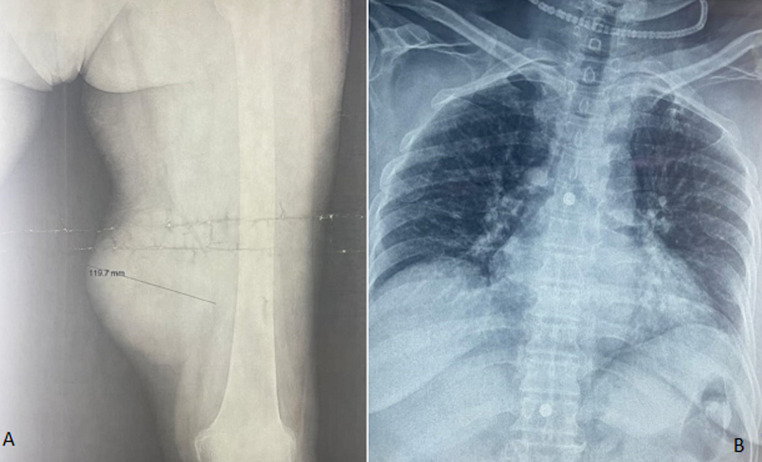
A) X-ray of the left thigh showing an oval opacity in the soft parts located in the inner face of the mid-thigh; B) chest X-ray showing pulmonary nodules of metastatic appearance
